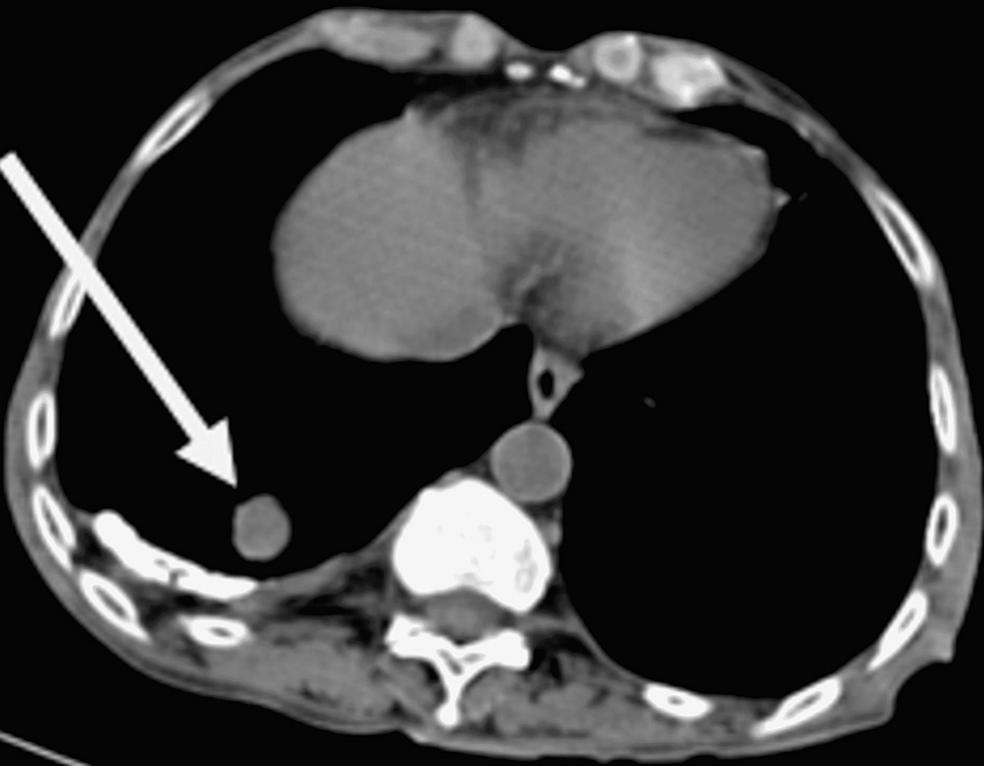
Non-contrast computed tomography scan showing lung metastasis with largest size of metastatic nodule measuring 17 x 18 mm in poster basal segment of right lower lobe.
